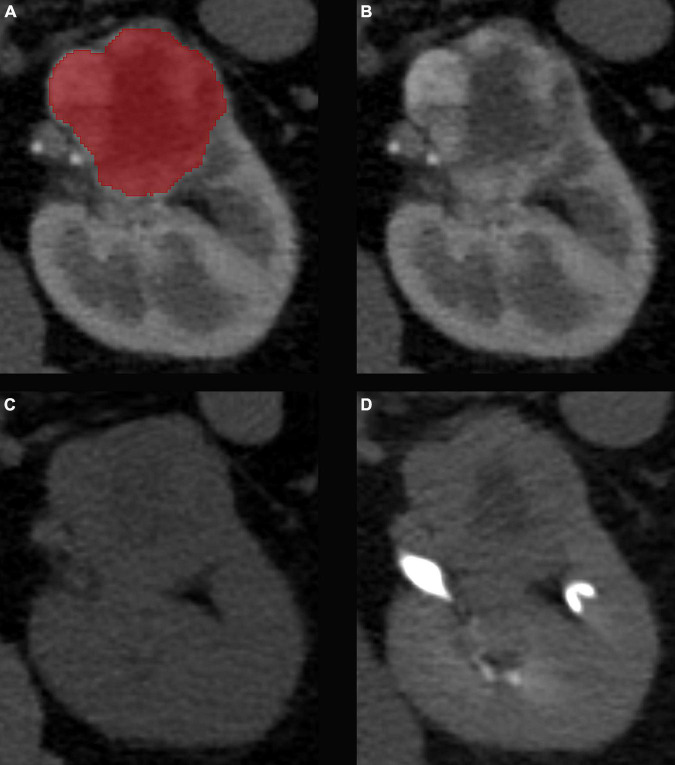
Results of the image co-registration. The manual segmentation of the kidney tumors was completed on the corticomedullary phase CT scans (A). A non-rigid image co-registration was performed to fit the unenhanced (B) and excretory (C) phase CT scans to the corticomedullary phase as reference (D).
